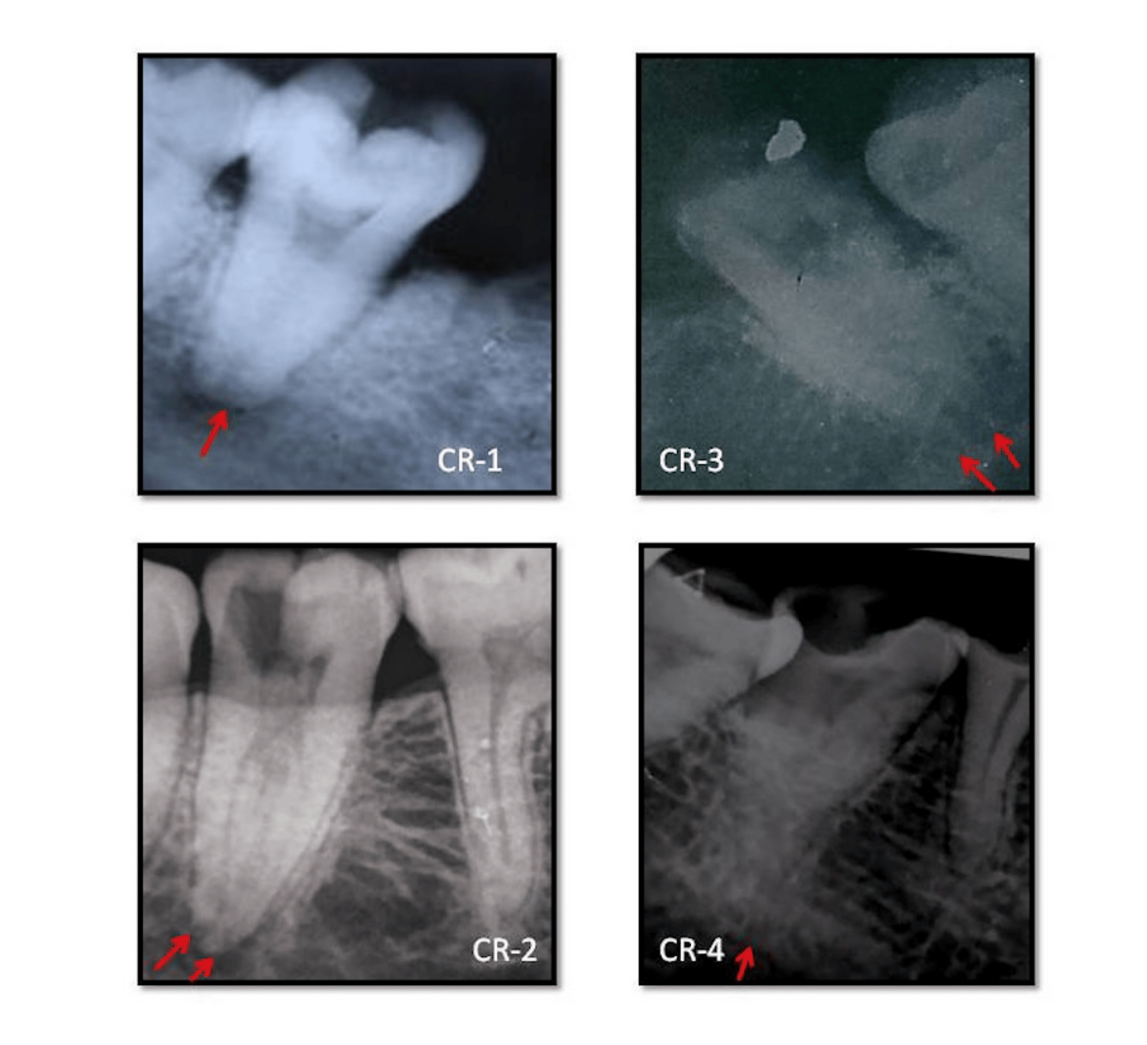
Preoperative radiographs CR-1: A single square shaped root; CR-2: Fused mesial and distal roots; CR-3: Fused mesial and distal roots; CR-4: A single conical root. CR: case report.
